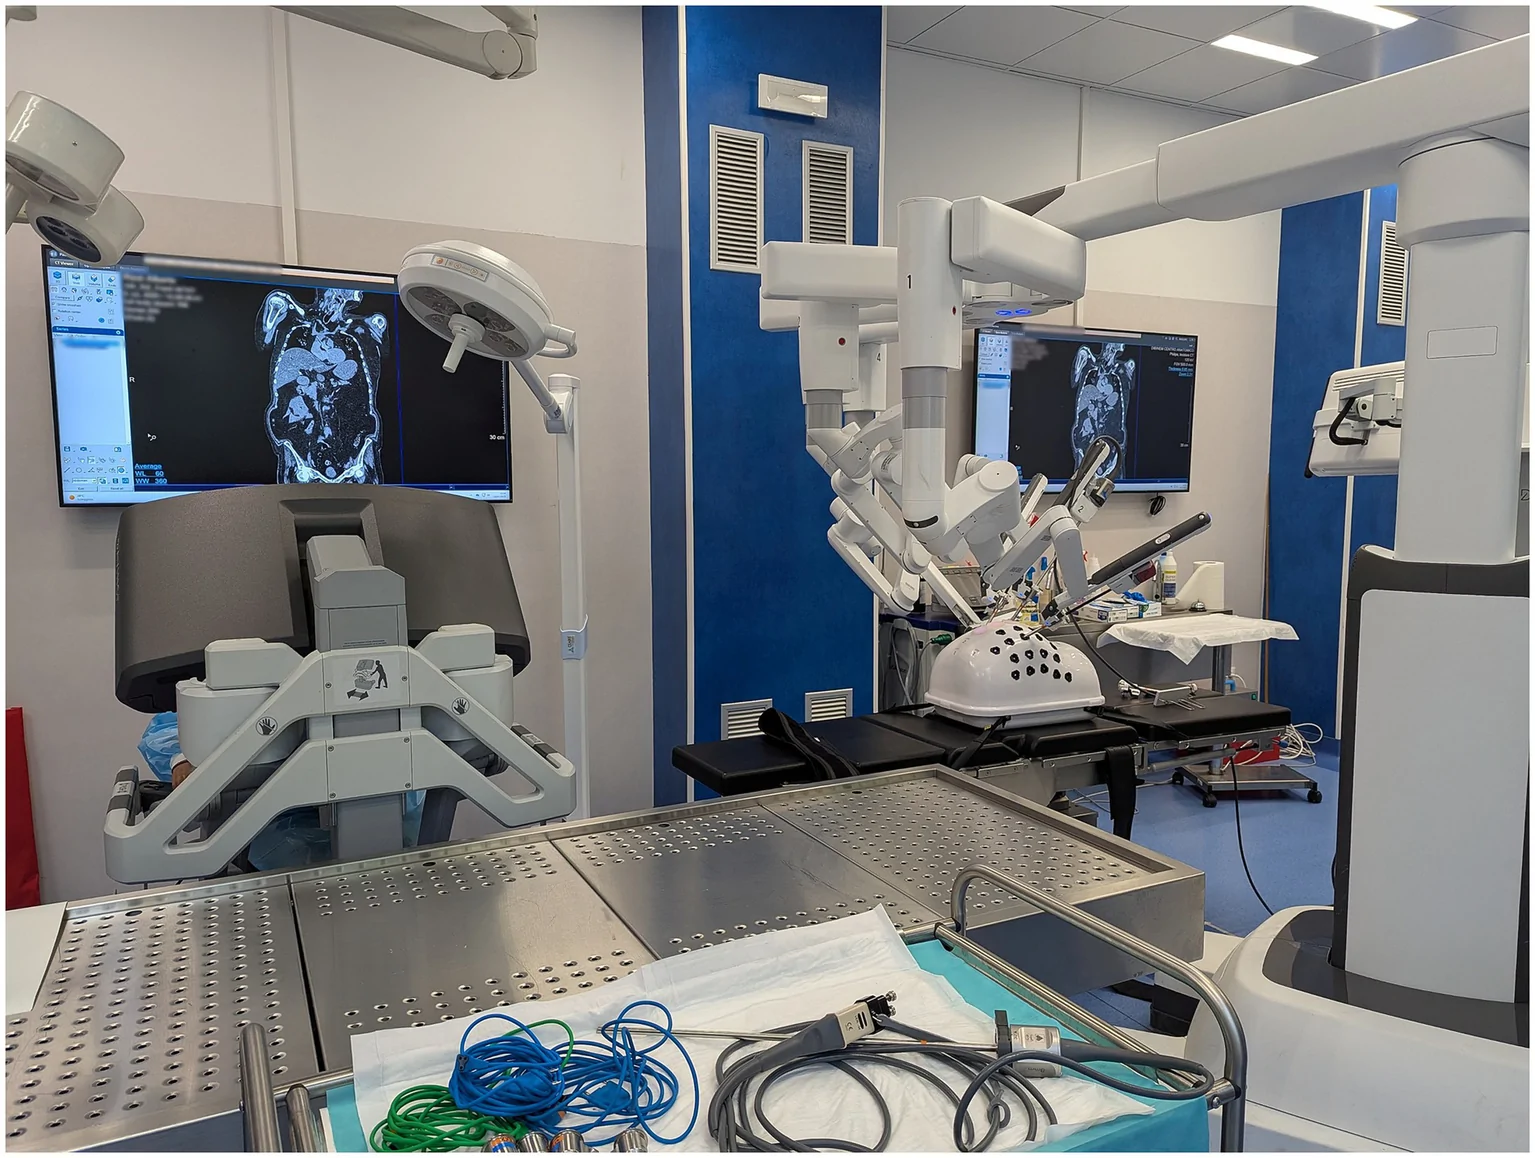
Dry-lab training set-up in the Mazzotti Room of the Anatomy Center of the University of Bologna. The da Vinci Xi patient cart is shown docked onto a pelvic trainer, with the room monitors displaying pelvic CT imaging in the background. The surgical console is visible on the left.
